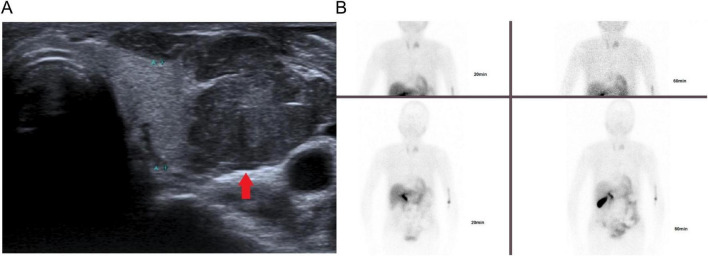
(A) Neck ultrasound revealed a heterogeneous hypoechoic mass (red arrow) measuring 26.6 × 20.4 mm located between the left thyroid lobe and the carotid artery. (B) Parathyroid 99mTc-MIBI scintigraphy: Early image (20 min): the thyroid is visualized in normal position and shape, and no obvious abnormalities are observed. The radioactive distribution is somewhat uneven. A circular radioactive uptake is seen in the middle part of the left lobe. No abnormal radioactive uptake is observed in the remaining neck or chest areas. Delayed image (60 min): in the images from different planes, the thyroid shadow appears weaker compared to the earlier image. The radioactive uptake in the middle part of the left lobe remains clearly visible.
